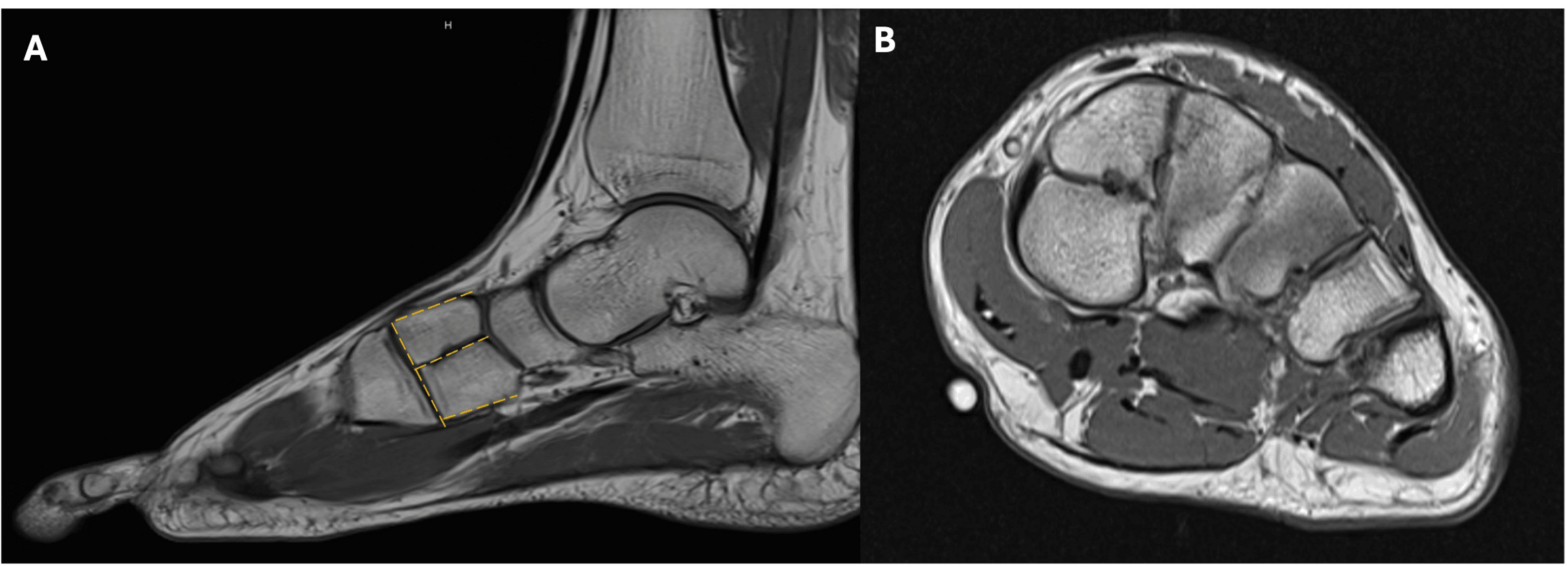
(A) Sagittal T1 MRI of the left foot showing bipartite medial cuneiform with characteristic "E" sign (yellow dashed line). (B) T1 short-axis MRI showing the appearance of bipartite medial cuneiform in short axis.
